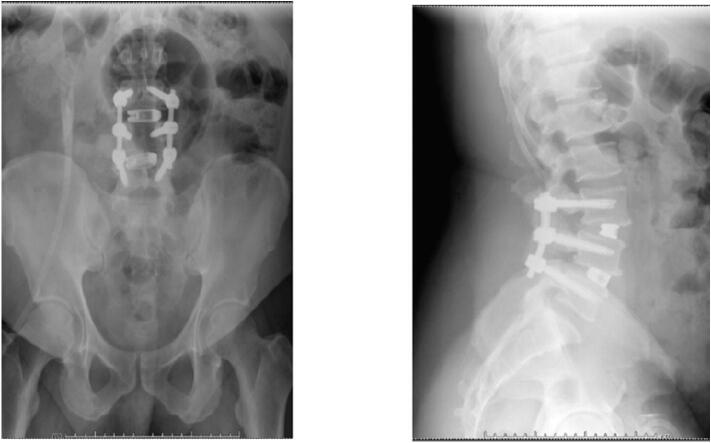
2/12/21- Postoperative Anterior/Posterior and Lateral Radiographs demonstrating intact L3–5 posterior decompression and instrumented fusion with L3–4, L4–5 transforaminal interbody fusion.
